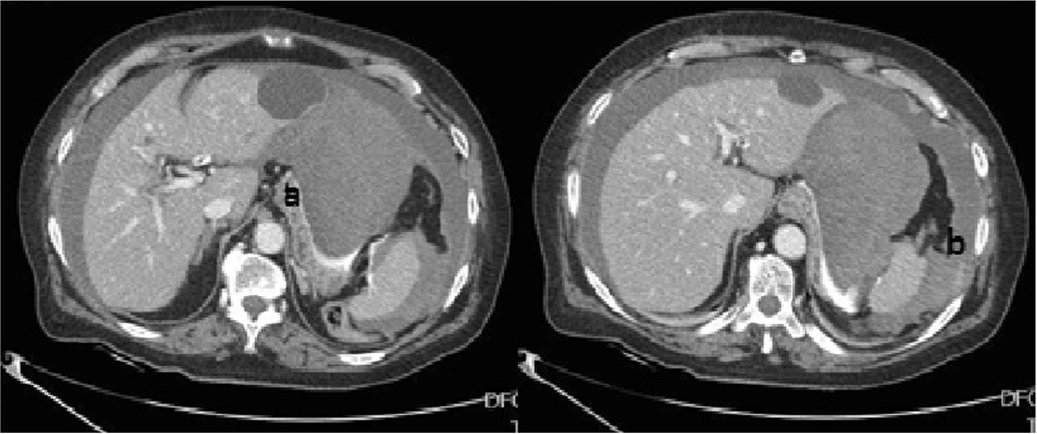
CT showing severe haemoperitoneum with two major blood collections localized respectively along the course of hepato-gastric ligament (a) and in the left sub-phrenic space (b).
